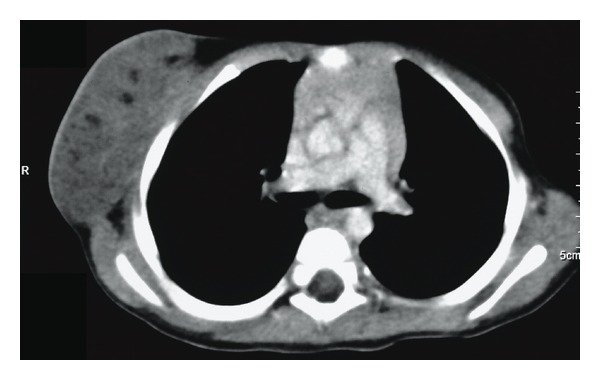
CT of the chest showing an 8 cm × 7 cm × 3 cm enhancing mixed density mass in the right chest wall.
